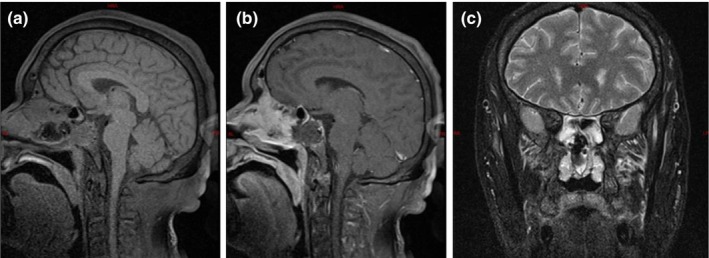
Postoperative reexamination of pituitary MRI shows normal morphology and uniform internal signal. No obvious abnormal enhancement area is observed. The shape of the pituitary stalk is normal and centered. There are irregular mixed signals in the left sphenoid sinus, with enhanced areas showing enhancement.
